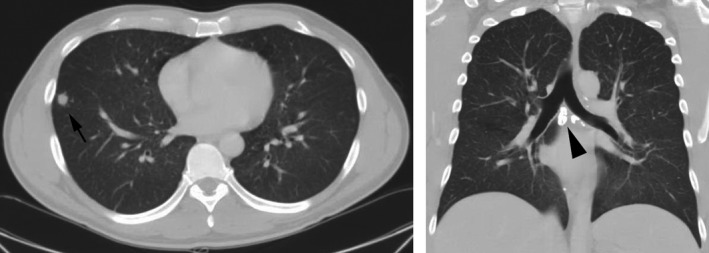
Chest computerized tomography (CT) scan with contrast showing numerous bilateral pulmonary nodules (arrow: 1‐cm nodule) and calcified mediastinal and hilar lymph nodes (arrowhead: mediastinal lymph nodes).
